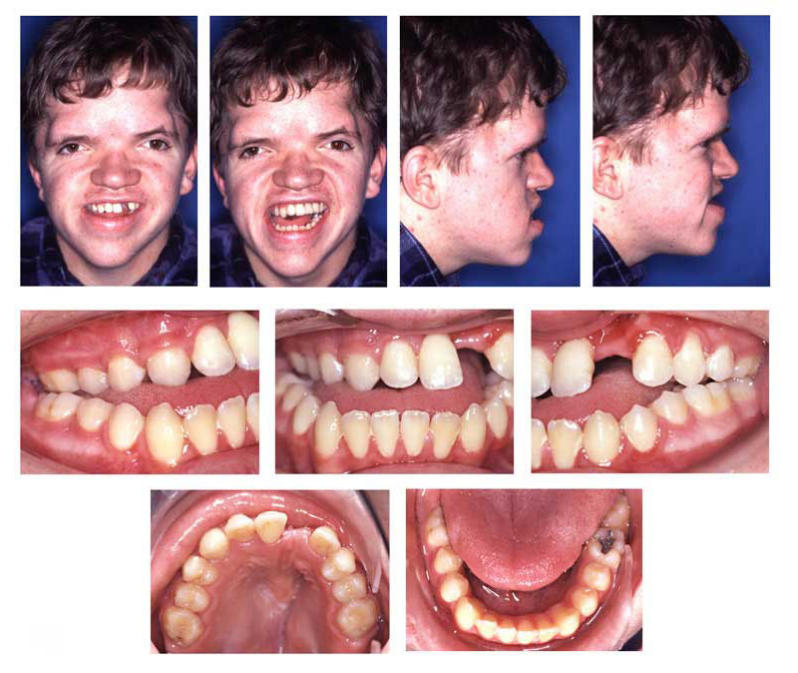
Age 16.4. Situation after 1.2 years in braces in order to parallelize 11 and 22 for later prosthodontic rehabilitation. Teeth 24 and 25 had not been bonded because of idiopathic root resorption (compare panographic x-ray, Fig. 16). Extraorally: With and without thermoplastic retainer for temporary replacement of 21. Intraorally: Teeth 11 and 22 were parallelized.
